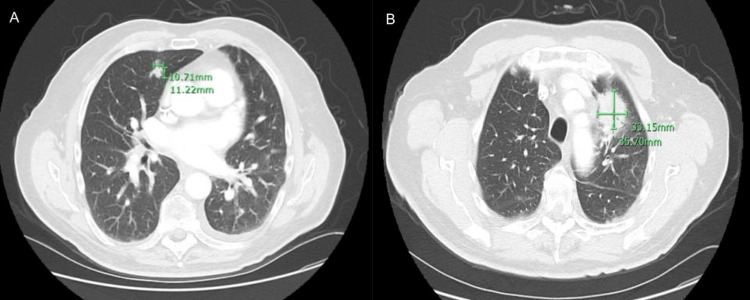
CT chest with contrast showed progression of the metastatic disease with an interval increase in the size of a right middle lobe nodule measuring 10.71 mm (A - axial view) and an interval increase in the size of a left upper lobe nodule at 33.15 x 35.70 mm (B - axial view).
